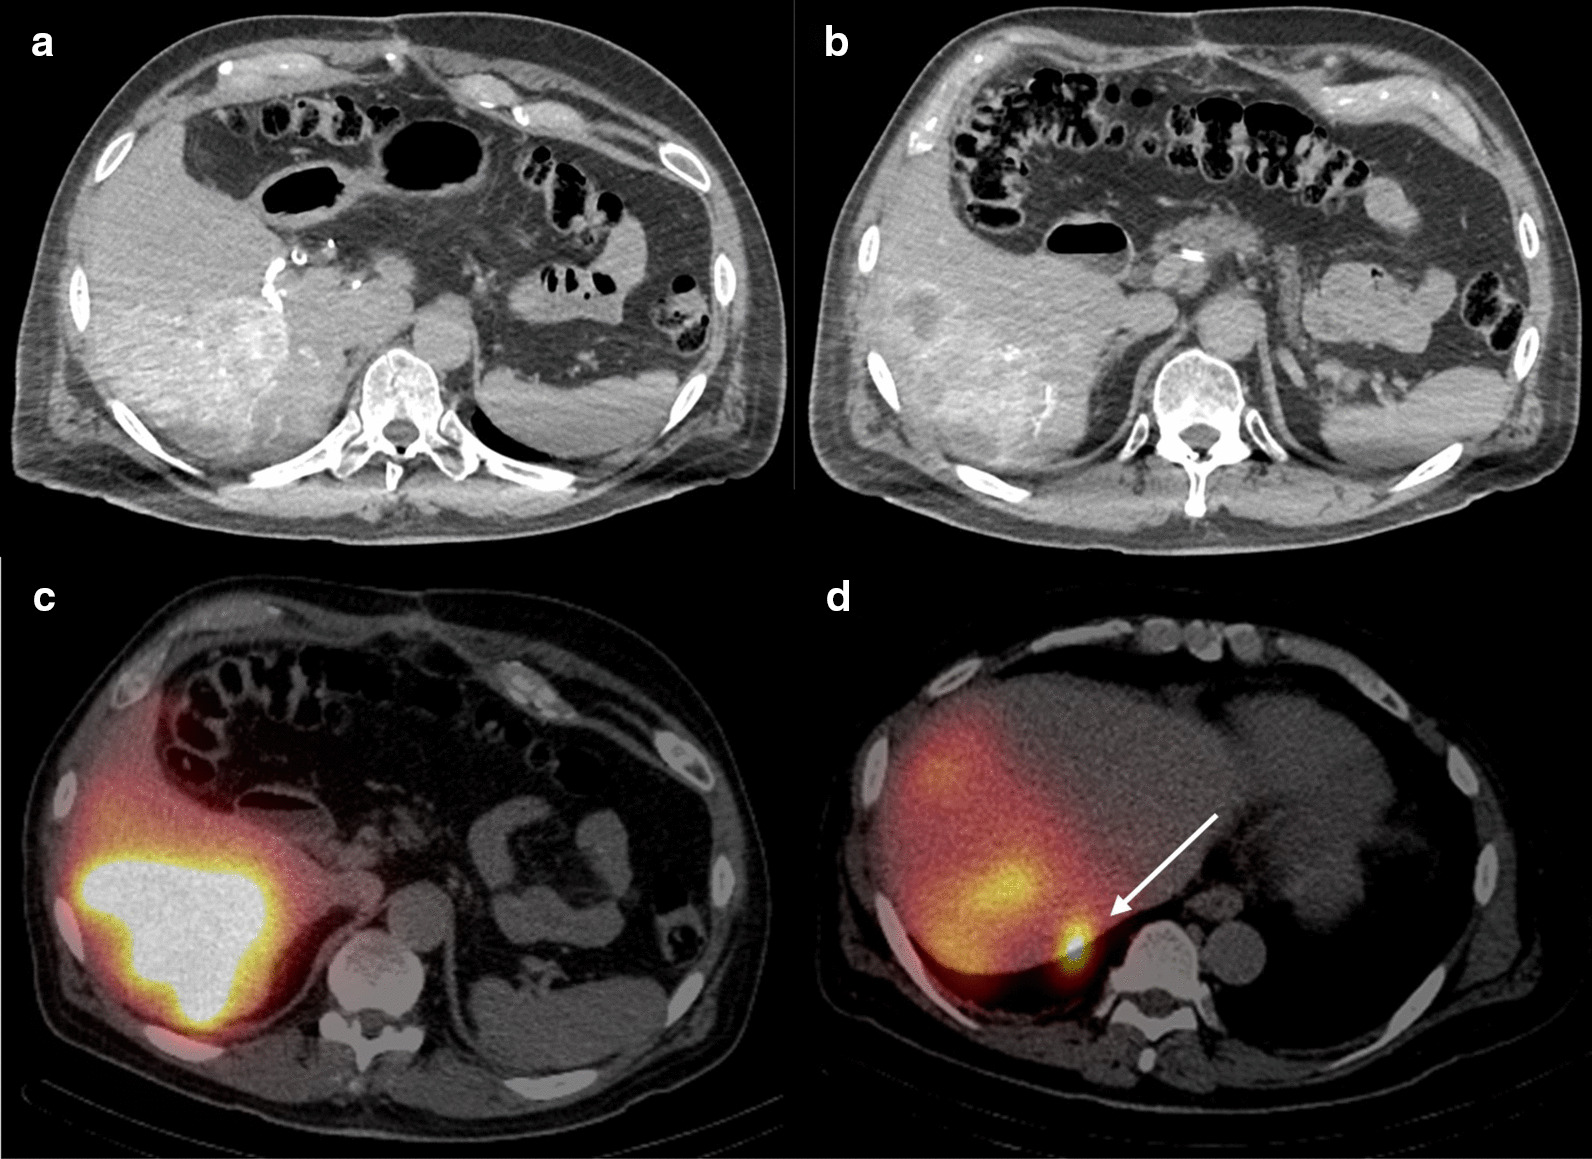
Axial images from pre ^90^Y 3D CT angiogram. a Intraprocedural selective arterial-phase contrast-enhanced CT of the right hepatic artery obtained during workup arteriography demonstrates hyperenhancing tumors with b hypoenhancing lesions along the posterior inferior liver, thought to be metastases supplied by branches of the right inferior phrenic artery. c SPECT/CT images after administration of [^99m^Tc]Tc-MAA into the right hepatic artery demonstrates intense activity within the majority of tumors in the inferior right hepatic lobe with photopenic peripheral areas that correlate to the hypoenhancing areas seen on 3D CT angiogram. d SPECT/CT images also demonstrate an area of intense focal activity along the posterior surface of hepatic segment VII (arrow), representing subserosal liver metastasis which may correspond to the plaque-like lesions seen during aborted initial surgery
